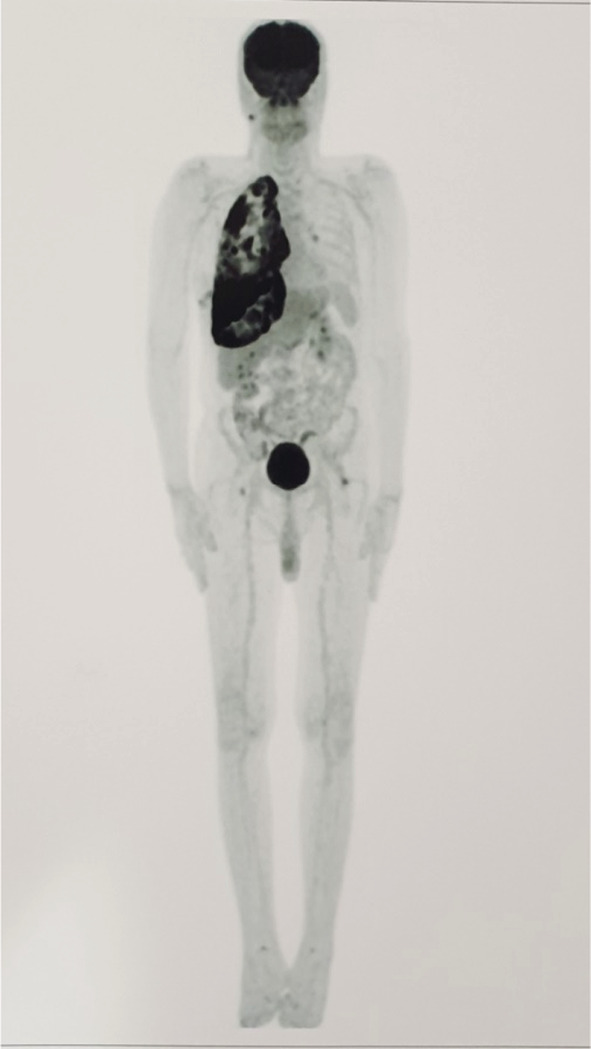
A hypermetabolic nodule in the right lower lobe, adjacent to oblique right fissure with SUV of 4.39 at maximum and hypermetabolic primary tumoral involvement in the right parietal and mediastinal pleura, along with malignant right effusion were noted. Diffuse irregular pleural thickening is noted on the right side, showing increased FDG activity (SUVmax: up to 7.98)
